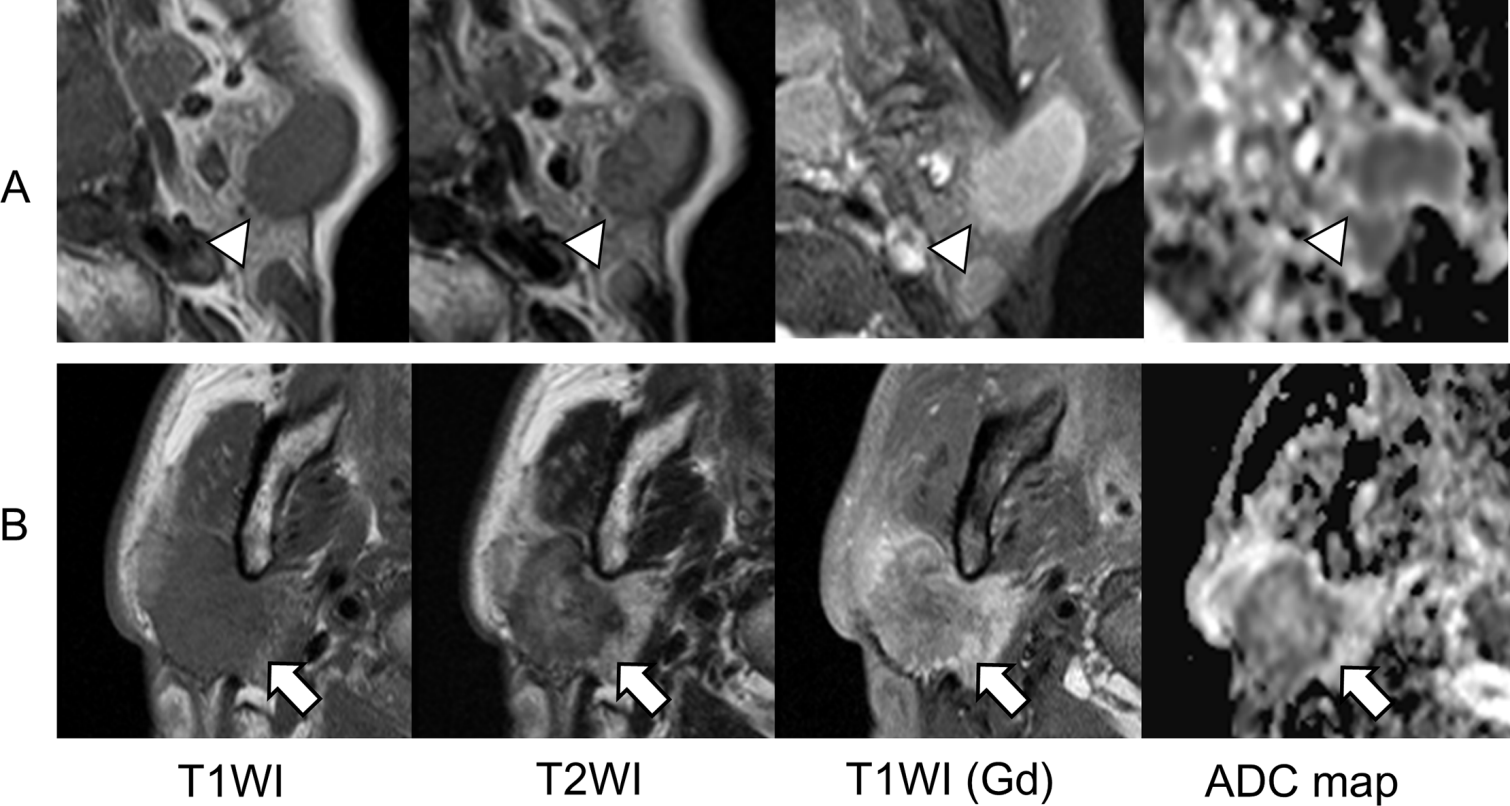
MRI findings in representative cases with malignant tumor. (A) Images of a diffuse large B cell lymphoma in the left parotid gland in a 48-year-old man (case 49). Axial MRI (white arrowheads) showed low intensity on T1WI, isointensity on T2WI, strong gadolinium enhancement, and a very low signal on the ADC map (ADC_mean_ 0.69 × 10^−3^ mm^2^/sec). (B) Images of basal cell carcinoma in the right parotid gland in a 69-year-old man (case 13). Axial MRI (white arrows) showed low intensity on T1WI, heterogenous (low to moderately high) intensity on T2WI, heterogenous gadolinium enhancement with poorly defined margins, and a low signal on the ADC map (ADC_mean_ 1.13 × 10^−3^ mm^2^/sec).
